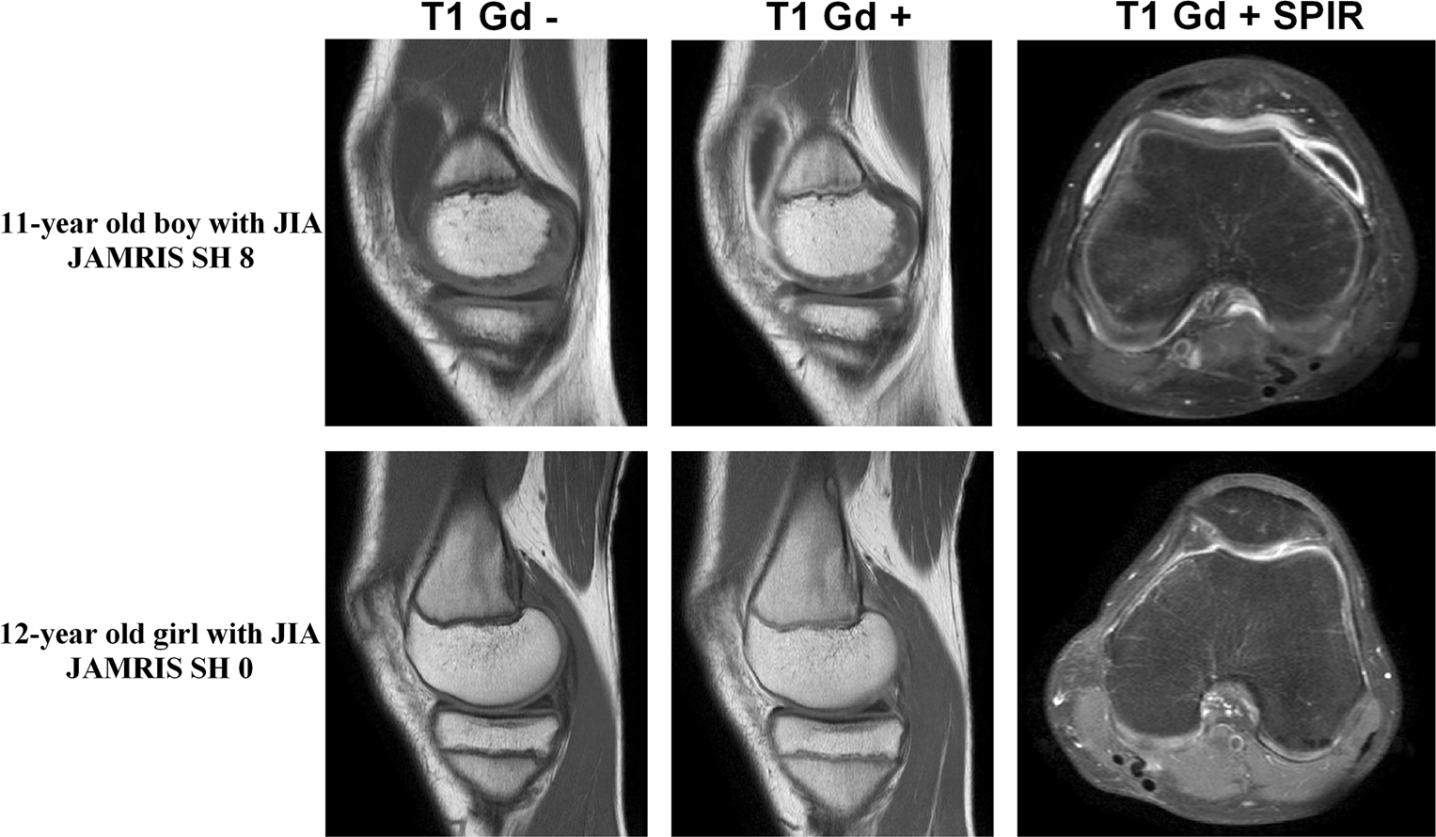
MRI example of two patients using a sagittal T1 pre- and post-contrast image and an axial T1 SPIR post-contrast image. MRI-active disease: the *upper row* represents an 11-year old boy with JIA who had synovial hypertrophy in multiple knee compartments, resulting in a total JAMRIS (Juvenile Arthritis MRI Scoring System) synovial hypertrophy (SH) score of 8. Note the enhancing and thickened synovial membrane in both the sagittal T1 post-contrast slice and the axial T1 SPIR. MRI-inactive disease: the *lower row* represents a 12-year old girl with JIA with no synovial hypertrophy in the knee (JAMRIS SH 0)
